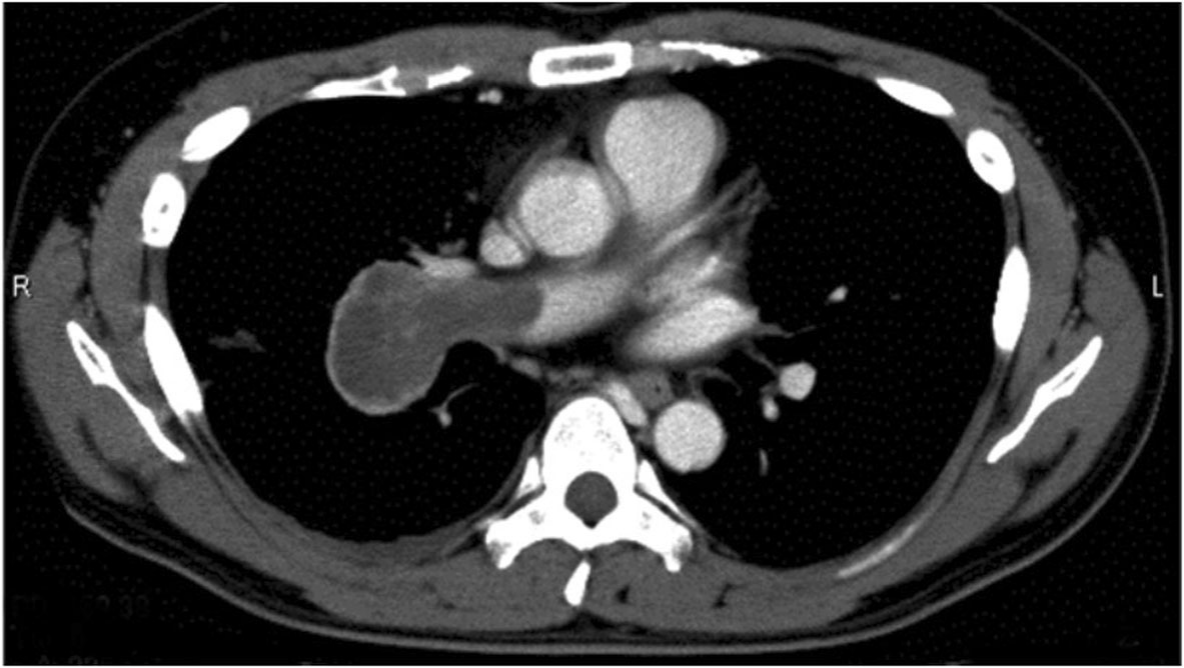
Contrast chest CT shows a right hilar mass that has invaded and occluded the right pulmonary artery
